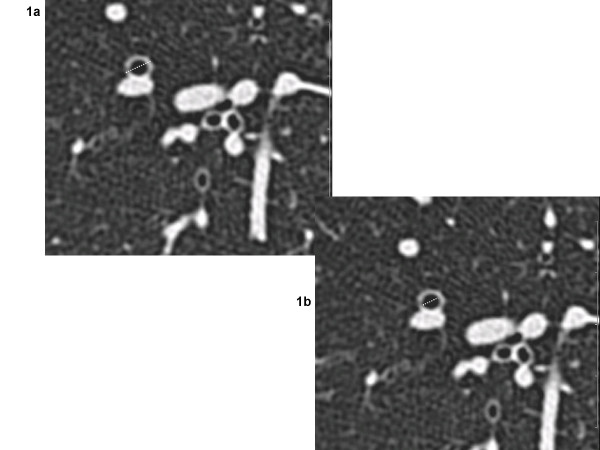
Outer and inner bronchial diameters. Magnified area of an axial HRCT of a child with difficult asthma showing a circular bronchus that was quantified. 1a) outer (Do = 0.5 cm) and 1b) inner (Di = 0.3 cm) bronchial diameters were measured as outlined.
